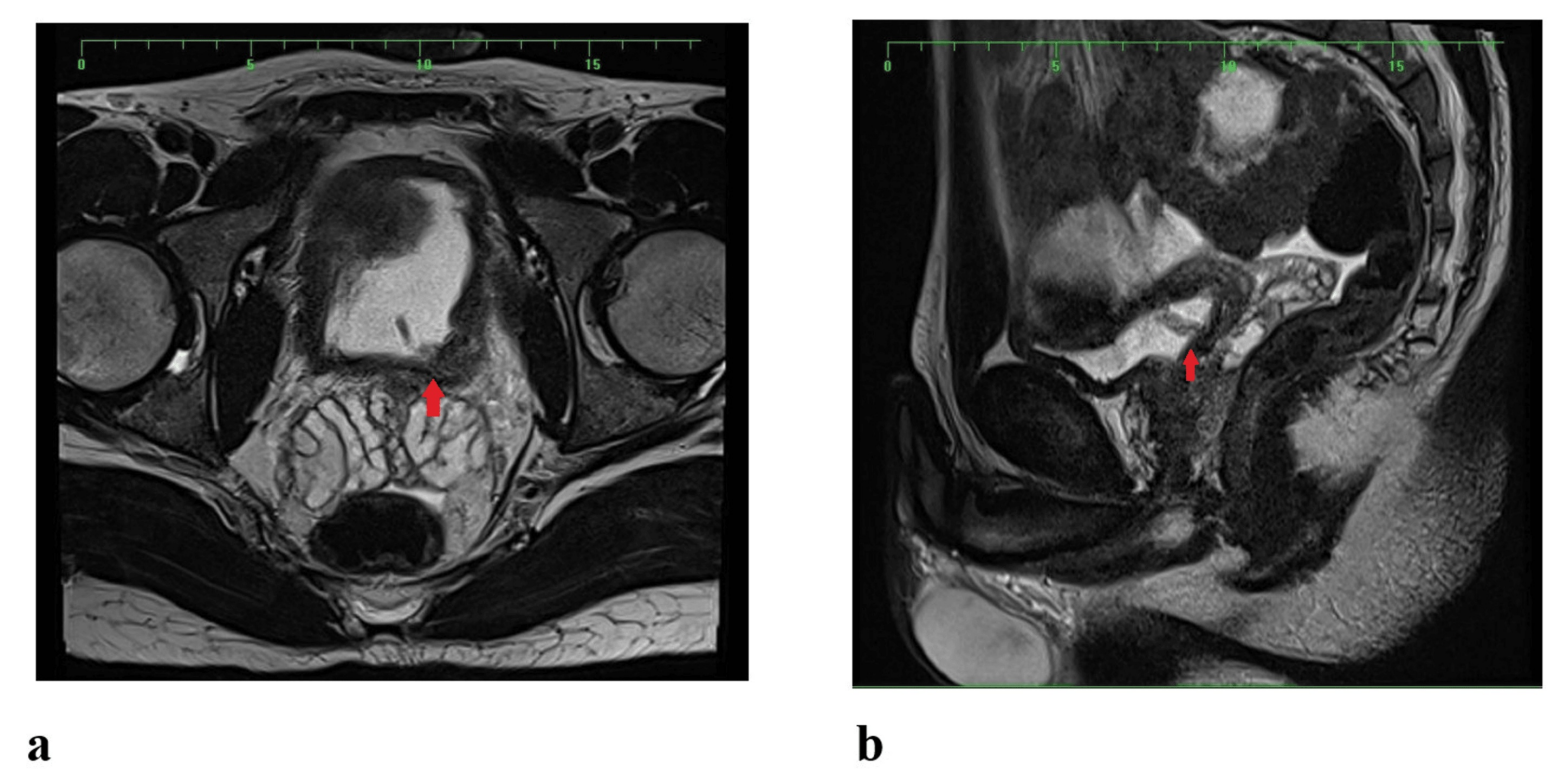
MRI showing possible extravesical involvement and loss of surrounding fat planes. Images taken in the (a) axial and (b) sagittal views, with arrows pointing to an enhancing, partially exophytic mass showing possible extravesical involvement and loss of surrounding fat planes.
